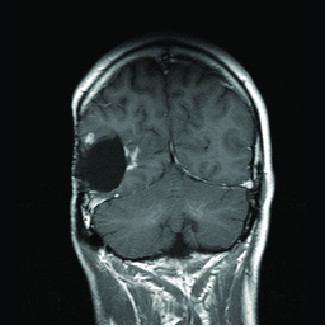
MRI obtained for treatment planning prior to fourth course of radiation therapy. Enhancement is noted adjacent to the surgical margin indicating progression of disease.
